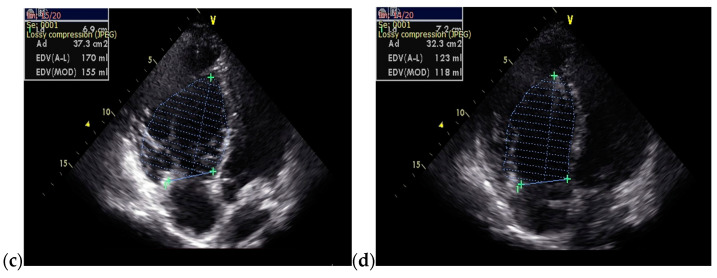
TTE on admission: (a) Apical 4-chamber view: dilated right cavities with a RV diameter of 7, 3 cm; (b) Apical 4-chamber view: total volume of the RV—252 mL; (c) Apical 4-chamber view: functional RV volume—170 mL; (d) Apical 4-chamber view: atrialized RV volume—123 mL; Abbreviations: TTE—transthoracic echocardiography; RV—right ventricle.
